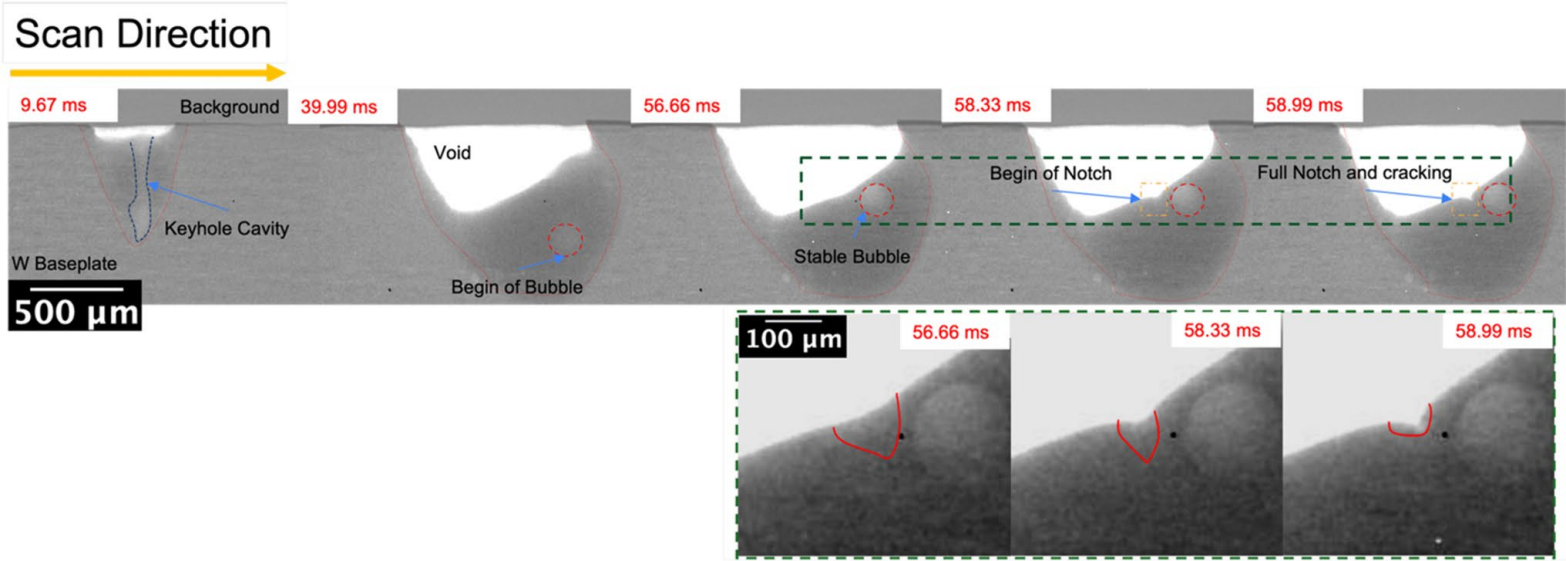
Selected images from a times series of high-energy synchrotron X-ray radiography for the laser conditions of 480 W and 25 mm/s. The images show the formation of a keyhole, bubble after the collapse of the keyhole , bubble travel to the top of the meltpool, notch initiation, and cracking. The images in the green box are higher magnification from the regions highlighted.
